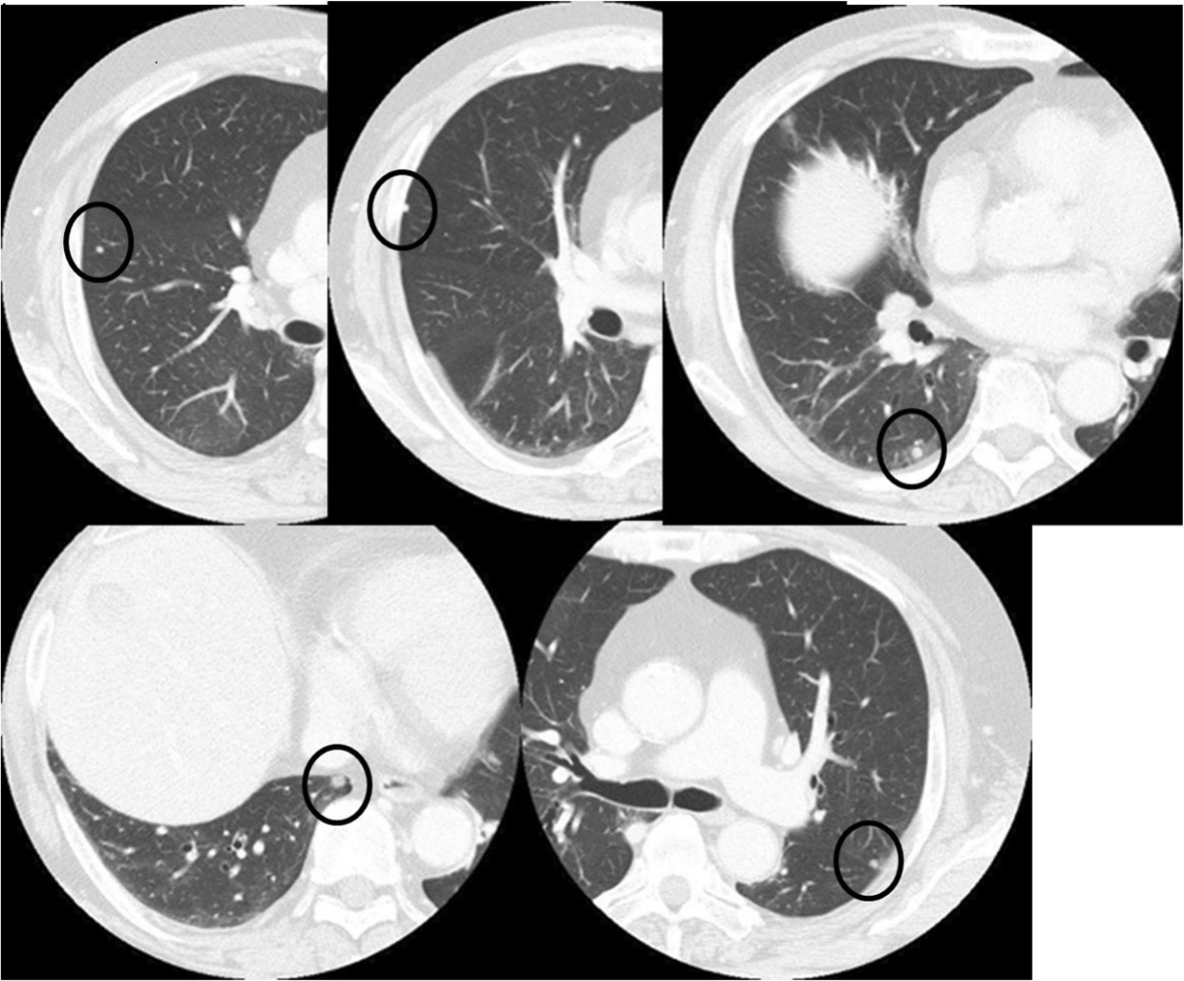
Chest CT 5 months after heavy ion radiotherapy. The chest CT shows multiple lung metastases 5 months after the heavy ion radiotherapy (*circles*)
